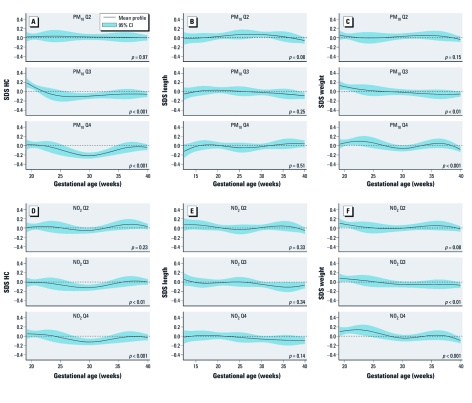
Associations of PM_10_ and NO_2_ exposure with longitudinally measured fetal growth characteristics. Figures are based on spline regression models of longitudinally measured (*A,D*) head circumference (HC) growth, 17,726 observations; (*B,E*) length growth, *n* = 20,305 observations; and (*C,F*) weight growth, *n* = 20,395 observations, all expressed in SD scores (SDS), for each quartile (Q) of air pollution exposure during pregnancy (from conception until delivery) compared with the reference group (lowest quartile). *A–C* present associations for PM_10_ exposure, and *D–F* present associations for NO_2_ exposure. Head circumference was estimated by ultrasound in second and third trimester of pregnancy and measured neonatally. Fetal length was measured by ultrasound as crown–rump length in first trimester and femur length in second and third trimester and as body length neonatally. Weight was estimated by ultrasound in second and third trimester of pregnancy and measured at birth. *p*-Values are based on multivariate *F*-tests and reflect the difference between the spline of each air pollution exposure category compared with the reference category. Models are adjusted for maternal age, body mass index, height, parity, ethnicity, education, folic acid supplementation use, smoking, alcohol consumption, noise exposure, paternal height, and fetal sex.
